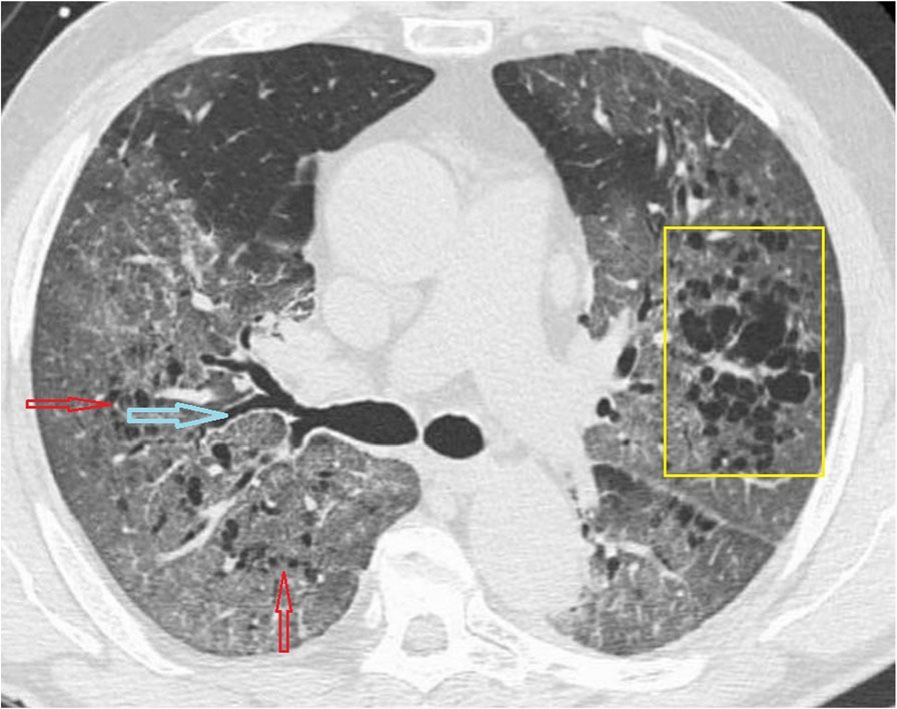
CT scan of COVID-19 patient shows reticular pattern superimposed on the background of GGO with air trapping at lower lobes (red arrow) as well as cystic changes at left lower lobe (yellow box). Bronchiolar irregularity at right lower lobe (blue arrow)
